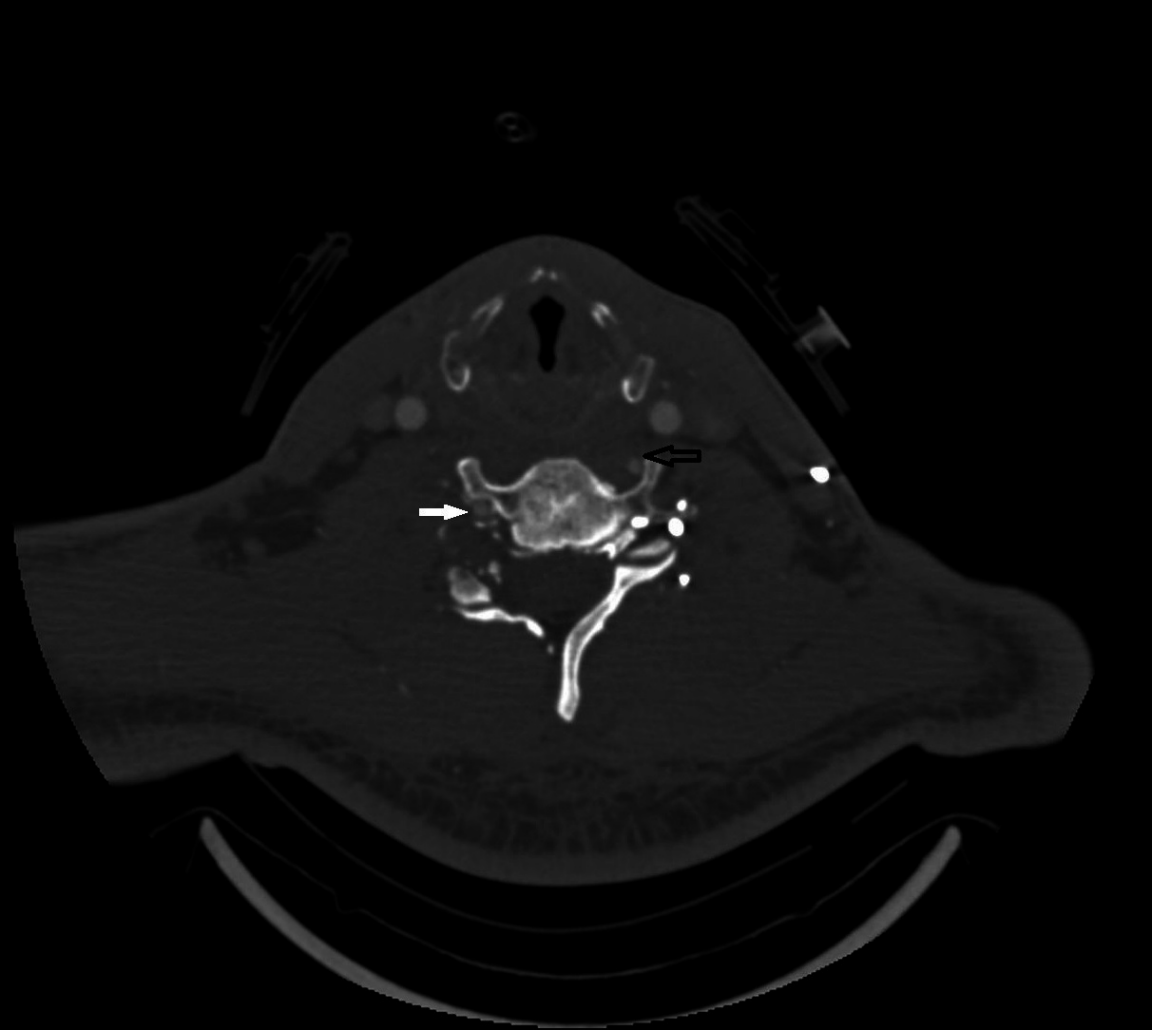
Computed Tomography Angiogram at C6 Computed tomography angiogram at the C6 level showing the vertebral artery in the foramen transversarium on the right (white arrow) and on the transverse process out of the foramen on the left (black arrow)
